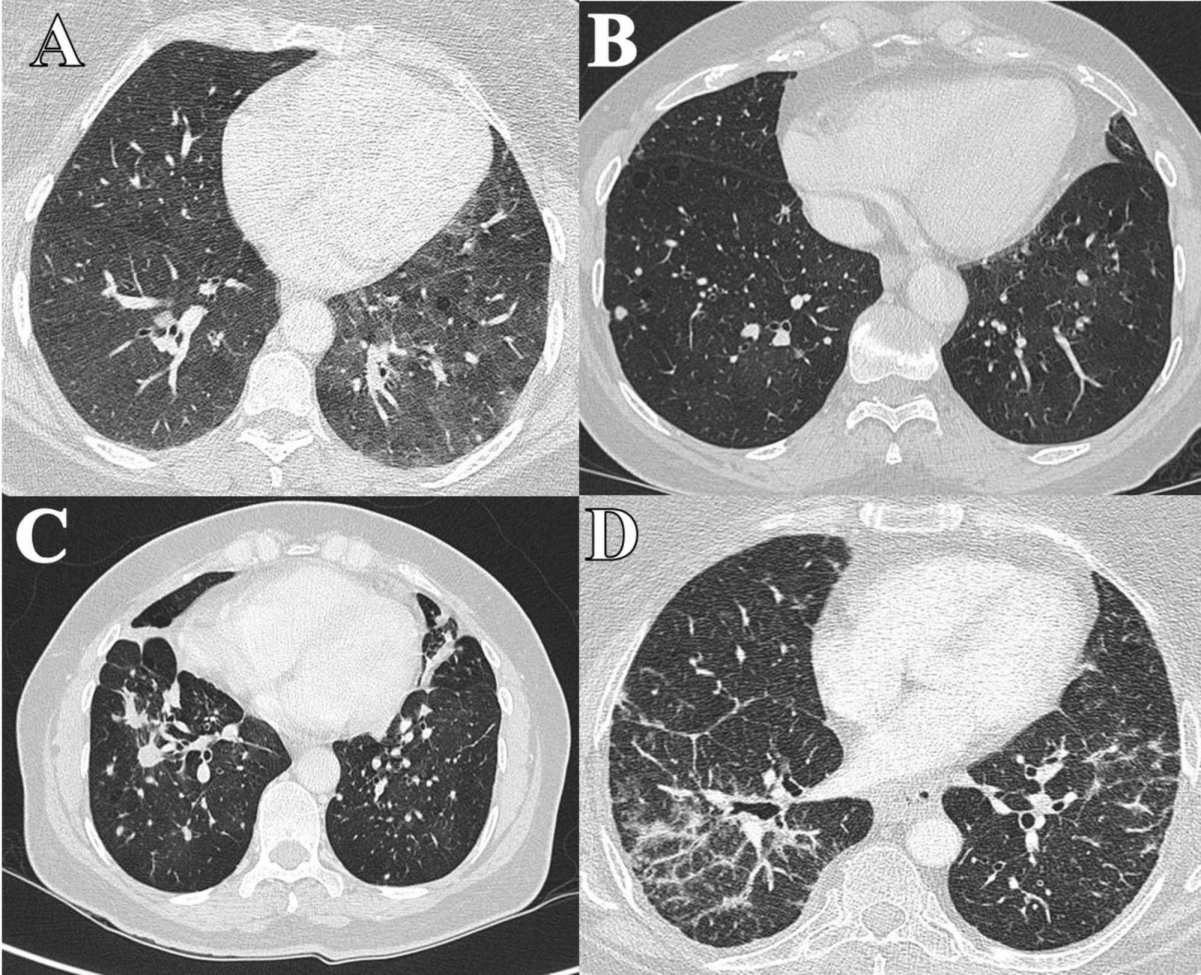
CT images (pulmonary parenchyma window). A (Case 1 patient). B (Case 2 patient). C (Case 3 patient). D (Case 4 patient).
